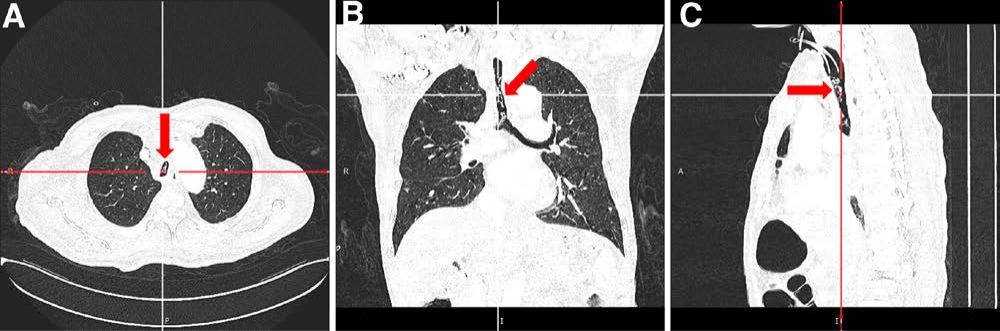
(A–C) Chest CT upon admission revealed multiple strip-shaped and columnar high-density lesions in the lumen of the trachea and the right and left main bronchi (red arrow). CT = computed tomography.
